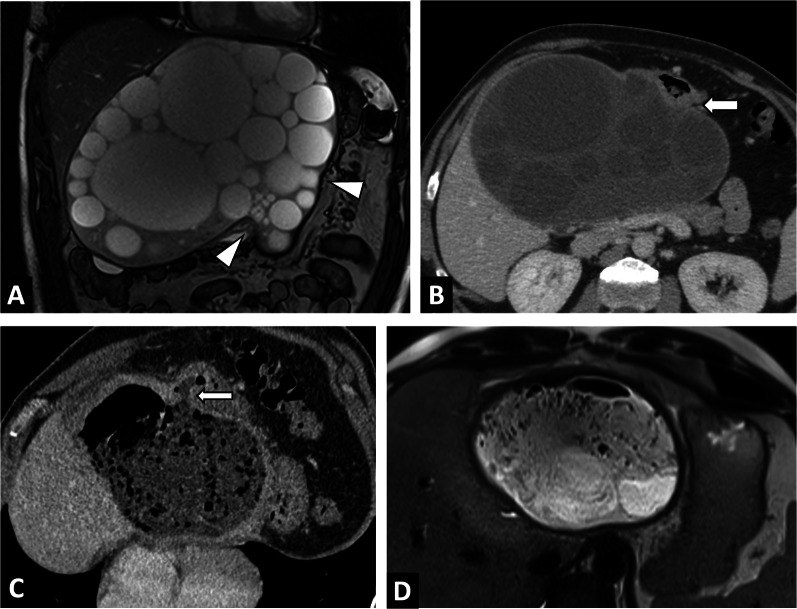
Large cystic echinococcosis lesion of the left liver at CE3b stage discovered during an investigation of abdominal pain. A T2-weighted MR image in the coronal plane: loss of continuity of the cyst wall (arrowheads) in contact with the gastric antrum. B CT image in the axial plane obtained during the portal phase of enhancement: close contact between the cyst wall and the gastric antrum (arrow). C CT image in the axial plane obtained during the portal phase in a context of fever and abdominal pain 3 months after the start of anti-parasitic treatment. Appearance of digestive content and air-fluid level within the CE lesion. Direct communication between the cyst and the gastric antrum (arrow). D Same lesion in T2-weighted MR image in the axial plane. Note the emptying of most daughter cysts, a sign of evacuation in the digestive tract
